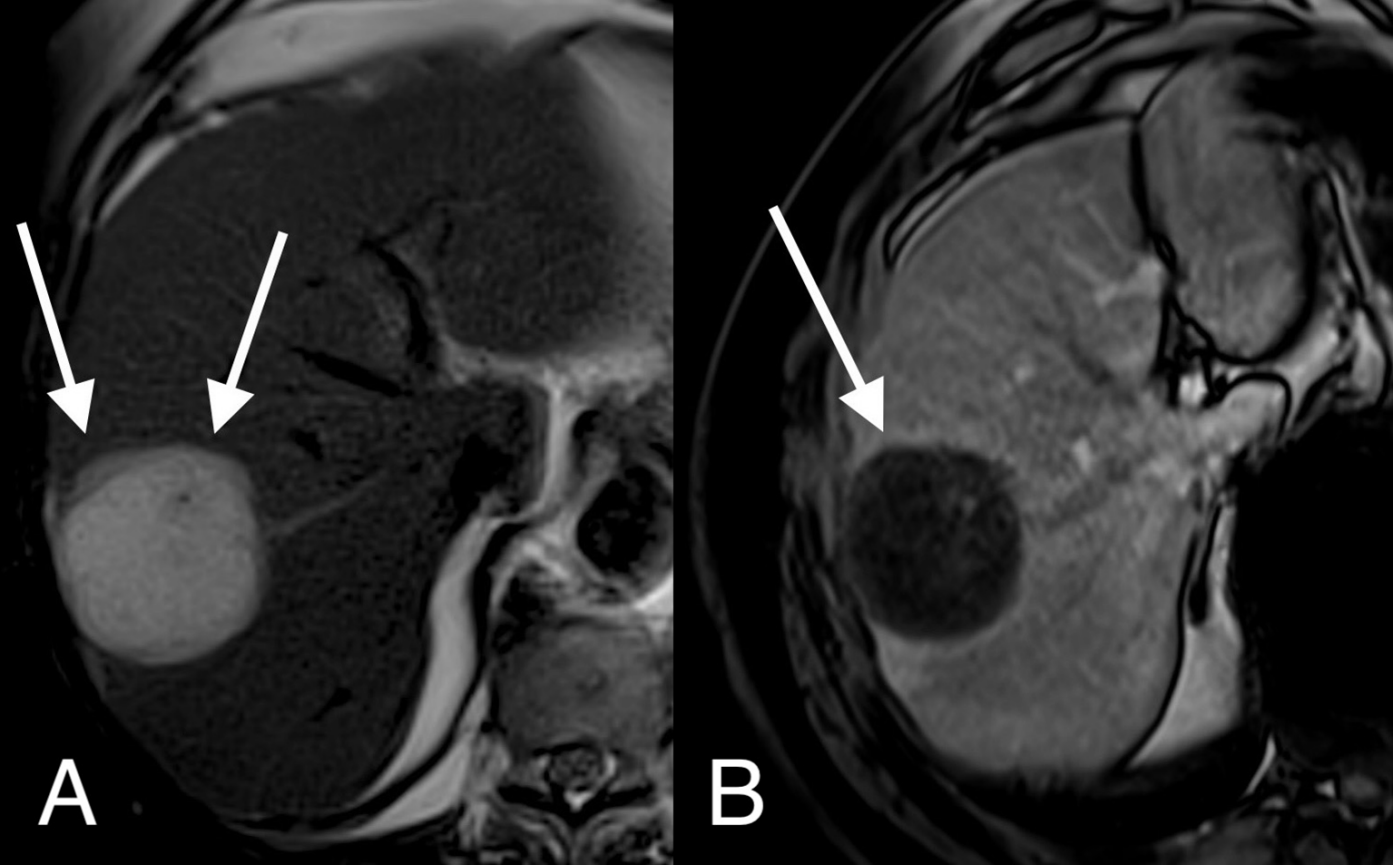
MRI image of a mucinous colorectal liver metastasis.Axial T2 weighted (A) and T1 weighted gadolinium enhanced (B) MRI images demonstrate a large T2 bright, cystic appearing lesion in segments 7 and 8 with a thick wall (arrows) and a thin rim of post-contrast enhancement.
